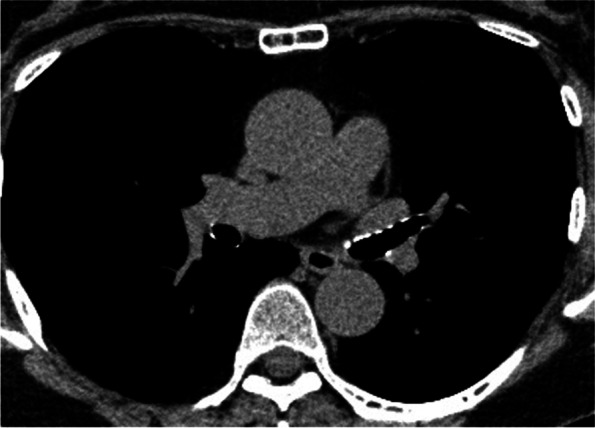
Chest CT imaging showed the stenosis markedly improved
